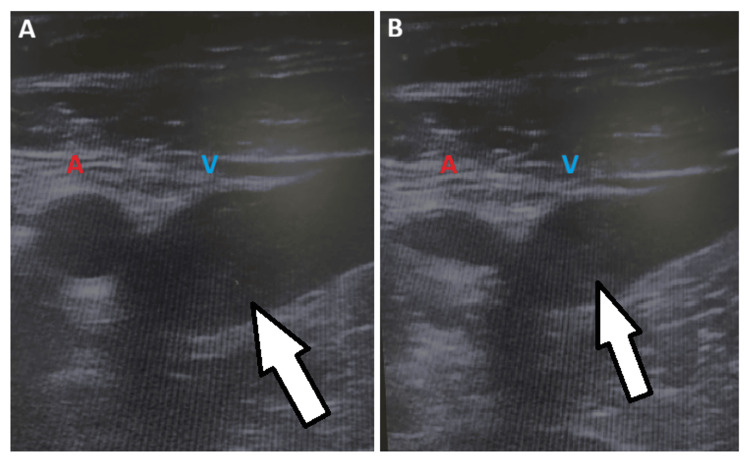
Right lower limb compression ultrasound showing DVT. Compression ultrasound of the right common femoral vein. The arrow indicates an intraluminal echogenic thrombus. A denotes the artery, and V denotes the vein. In panel A (before compression), both vessels are visualized. In panel B (during compression), the artery collapses normally, while the vein remains non-compressible, consistent with deep vein thrombosis. DVT: deep vein thrombosis
